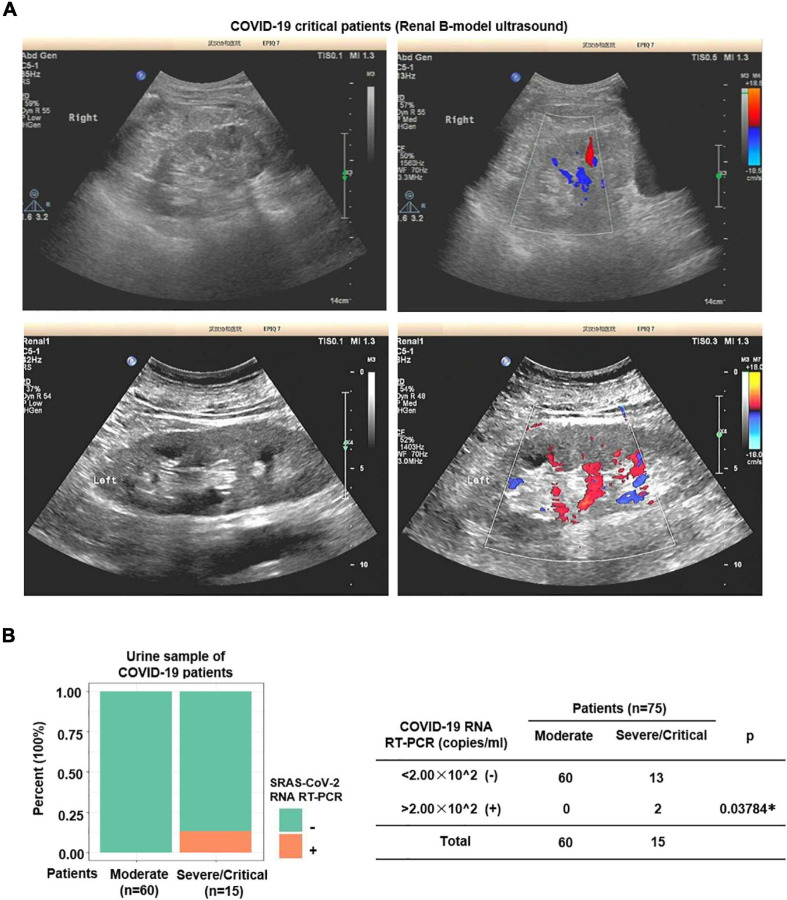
Nucleic acid of SARS-CoV-2 was detected in urine of severe patients. (A) Color Doppler imaging showed reduced perfusion in severe COVID-19 patients. (B) SARS-CoV-2 RNA Real-Time PCR was performed on urine samples. SARS-CoV-2 nucleic acid was detected in 2 out of 15 (13.3%) severe samples, whereas none of the moderate samples (*n* = 60) was positive.
